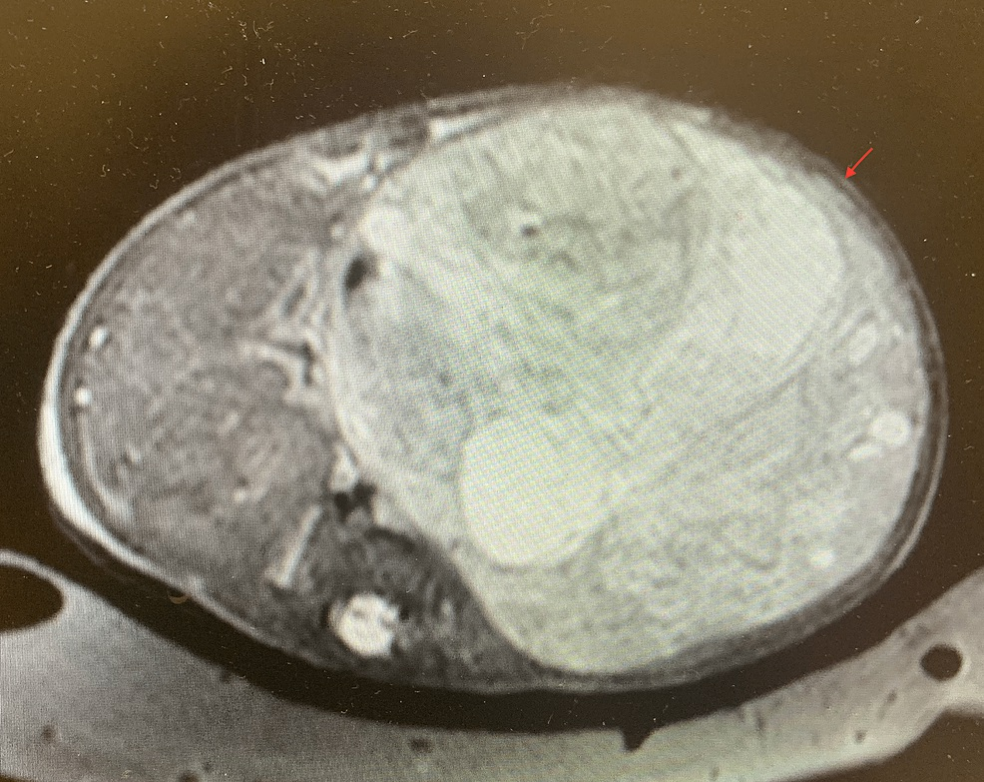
Abdominal MRI axial image displaying the large tumor (arrow) MRI: magnetic resonance imaging
